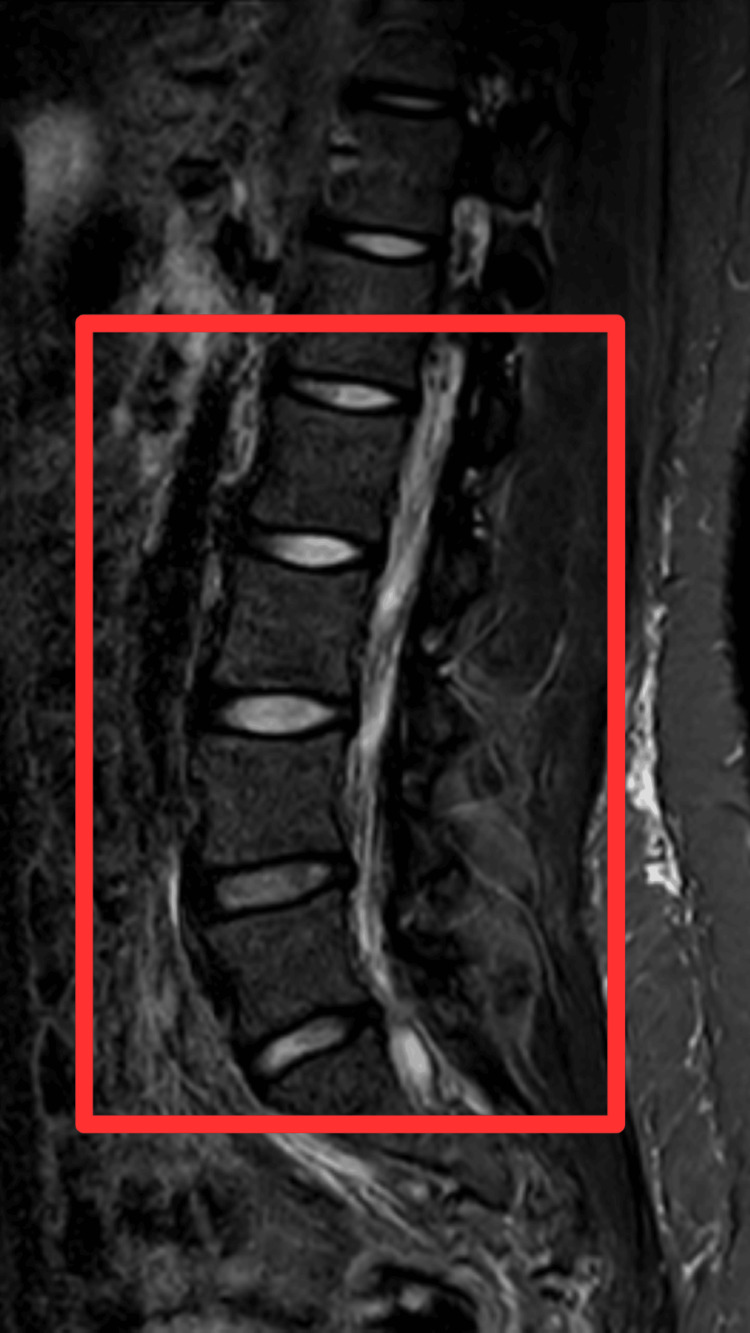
MRI findings of patient MRI findings show mild asymmetrical disc bulge at L1-2 level and mild disc bulge at L2-3 level, disc desiccation, and diffuse circumferential disc bulge causing mild bilateral neural foraminal narrowing at L3-4 level, it indents anterior thecal sac and abuts bilateral traversing L4 nerve roots, mild decreased disc height, and diffuse circumferential disc.
